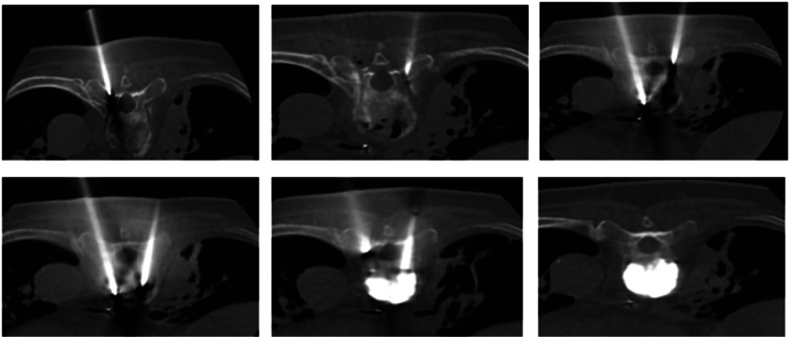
Axial CT imaging of bilateral transpedicular approach.
